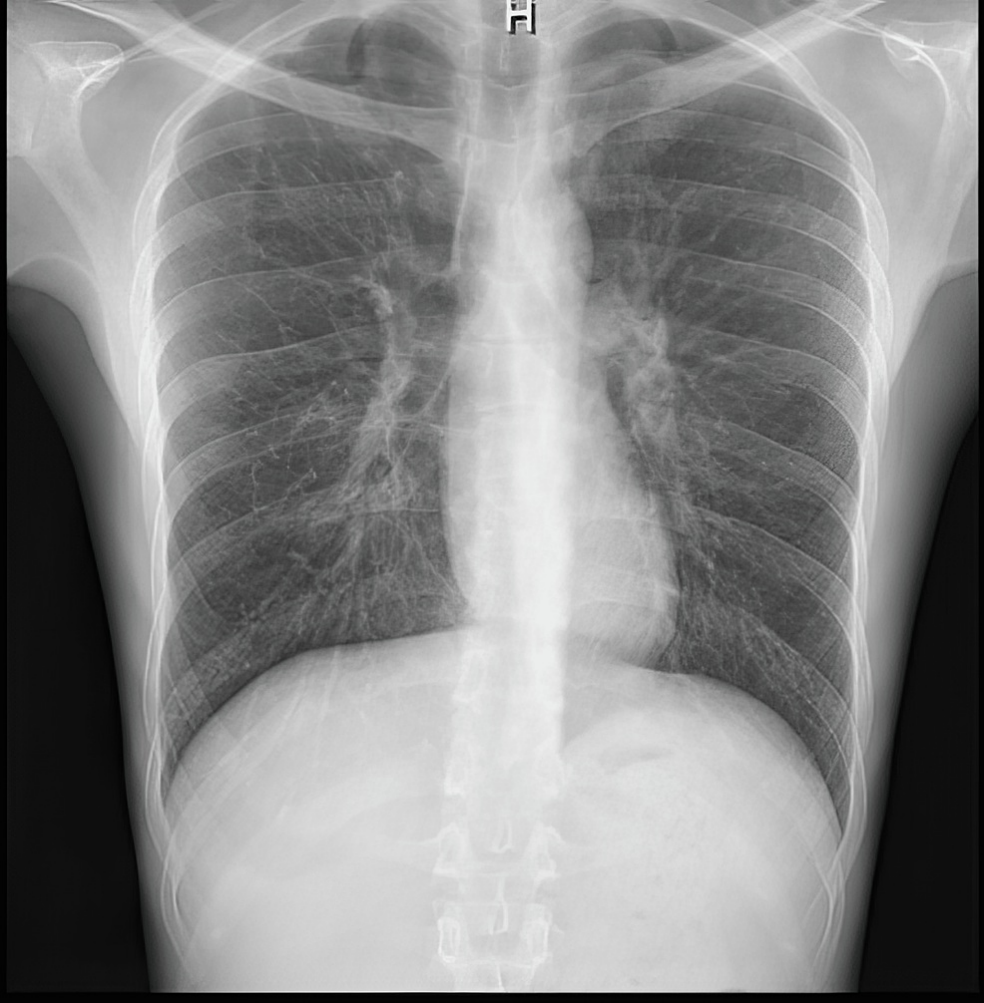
Repeat chest X-ray after a few days into treatment with steroids The X-ray image is showing a complete resolution of lung infiltrates.
